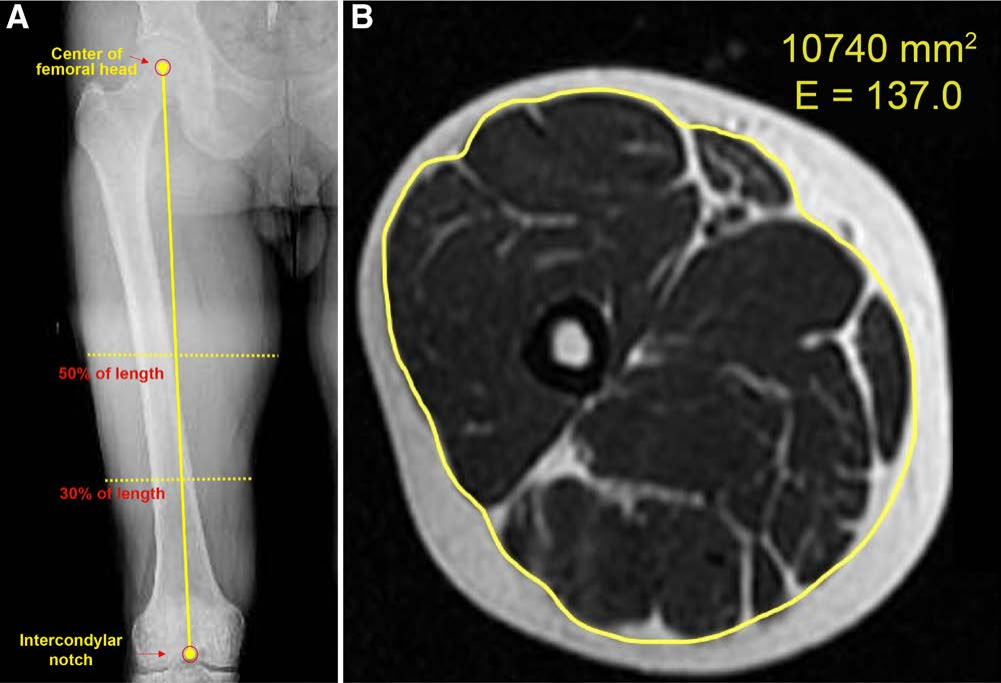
(A) Confirmation of the measurement of the thigh cross-sectional area location using full-length X-ray. (B) Measurement of the cross-sectional area of the thigh at 50% of the distal femur with the free ROI technique in the PACS program. ROI = region of interest.
